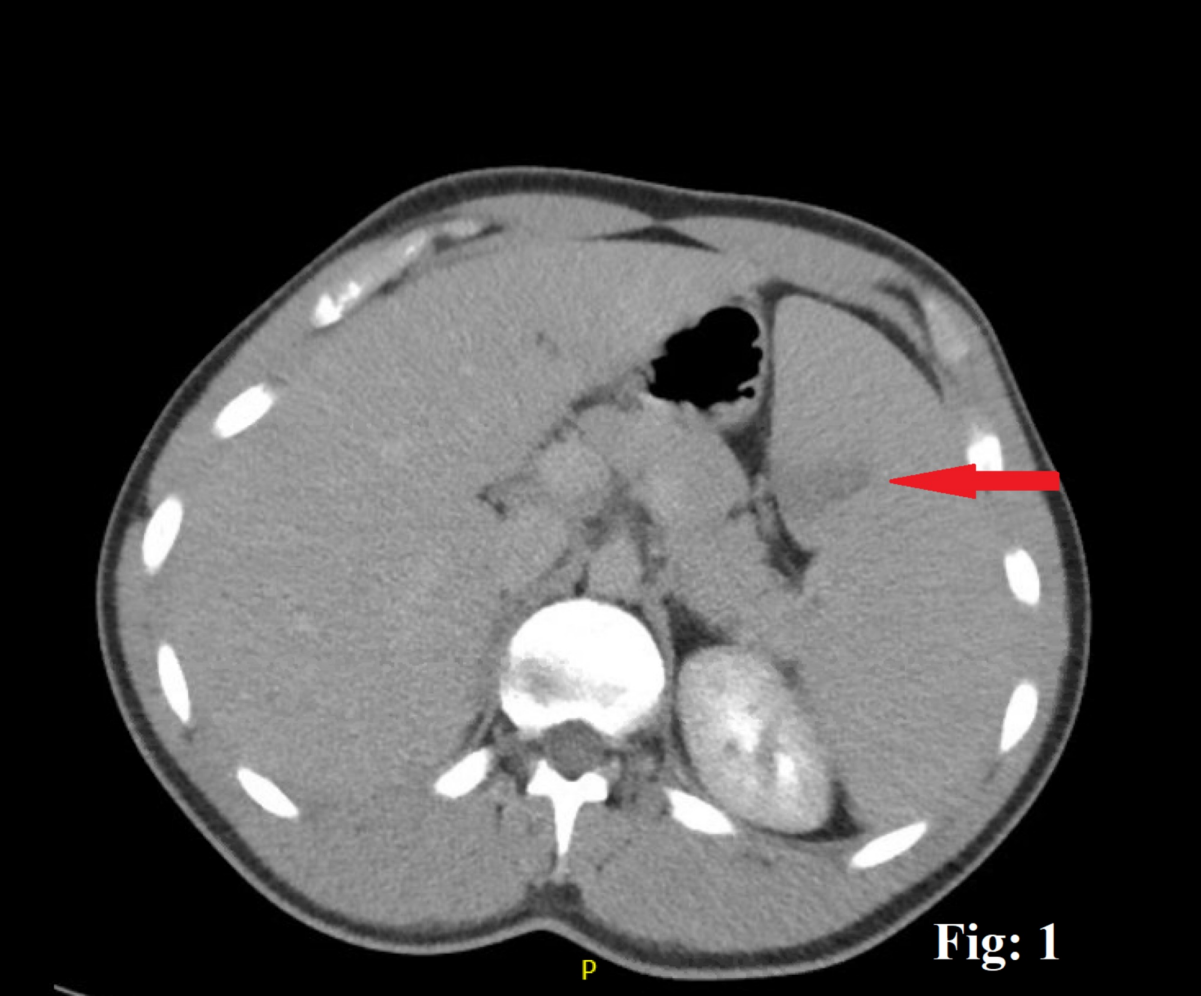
A contrast-enhanced computed tomograpghy scan of the abdomen, the red arrow showing wedge-shaped splenic infarct.
